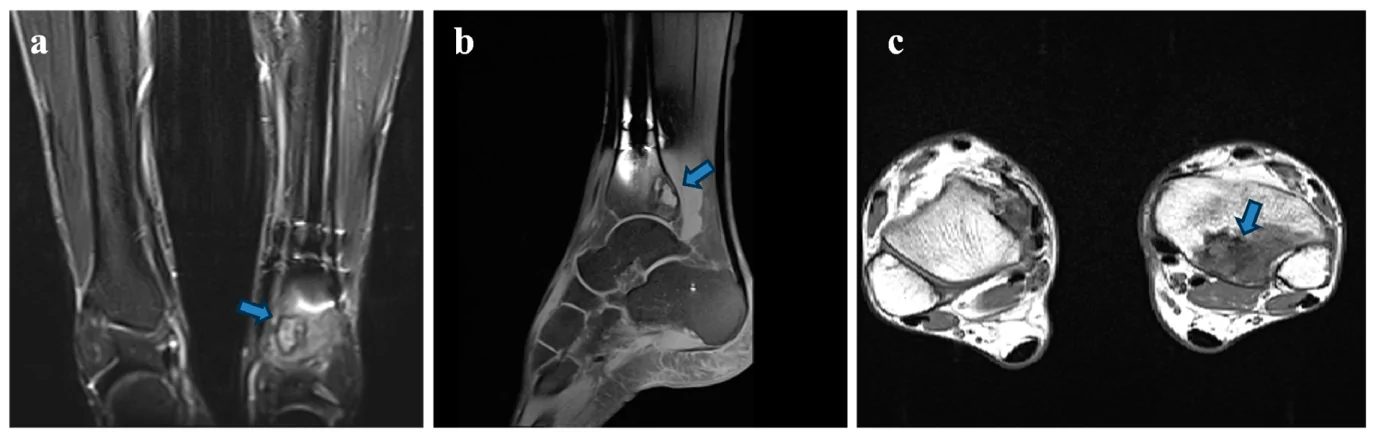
Contrast-enhanced MRI performed in April 2021 demonstrating the lesion (blue arrows): post-contrast enhancement on the coronal plane (a), features suggestive of high cellularity on Dixon sequences in the sagittal plane (b), and a poorly defined lesion with low signal intensity on T1-weighted axial images compared with the contralateral distal leg (c).
